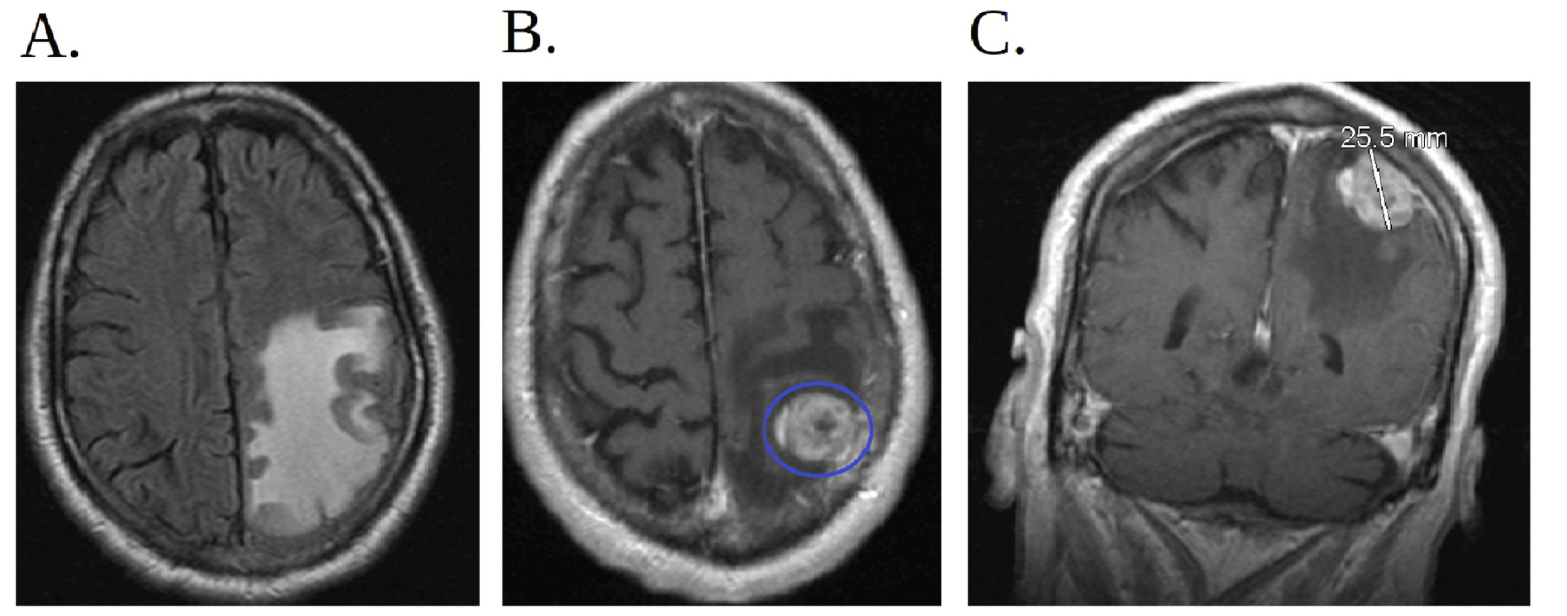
Repeat MRI of the brain and head with and without contrast (A) The figure is a transverse view of the MRI of the brain with and without contrast which was done two months later and shows worsening prominent adjacent vasogenic edema. (B) The blue circle indicates an increasing size of a left parietal cortical/subcortical mass now measuring 2.6×1.9×2.2 cm with increasing vasogenic edema involving the left frontal and parietal lobes which was done two months later from the initial MRI of the brain. (C) The figure is a coronal view of the MRI of the brain with and without contrast which was done two months later from the first MRI of the brain and shows the coronal view of the left parietal cortical/subcortical mass now measuring 2.6×1.9×2.2 cm. MRI: magnetic resonance imaging
